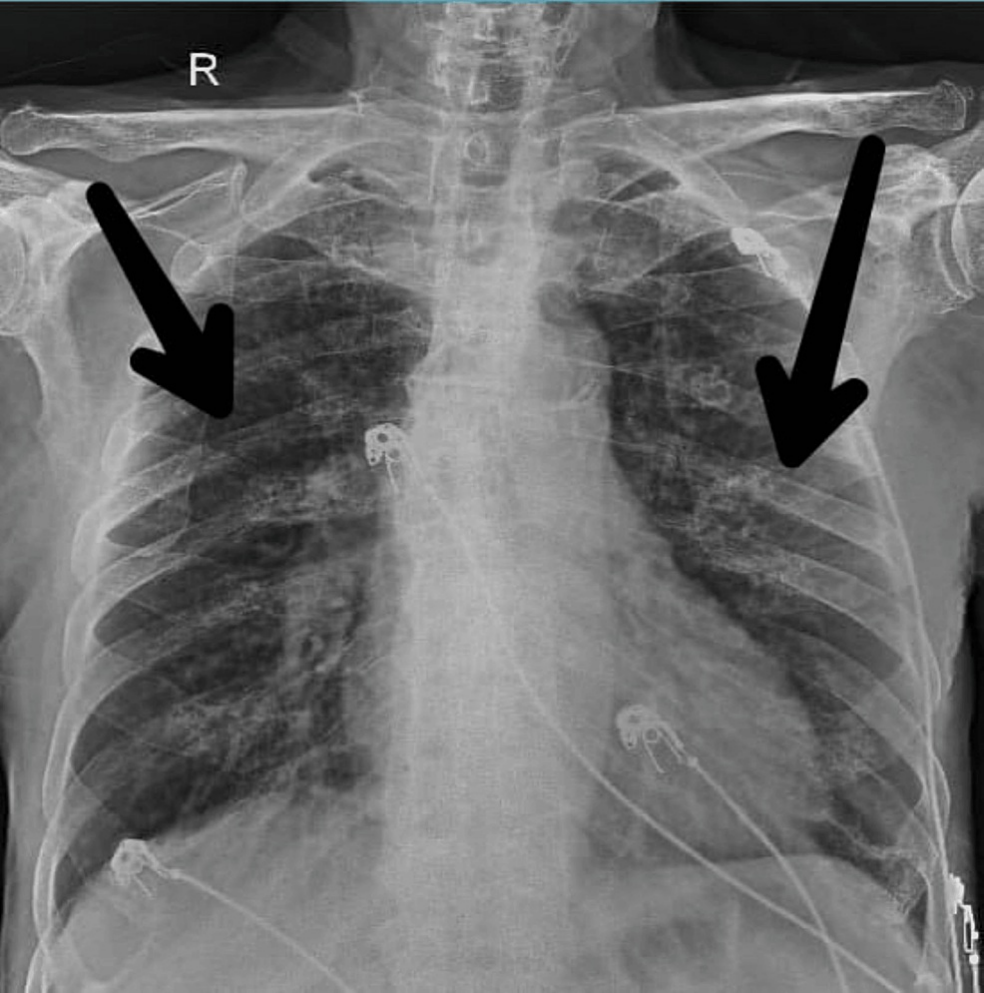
Chest X-ray in PA view showing relatively clear lungs post intervention PA: posteroanterior
